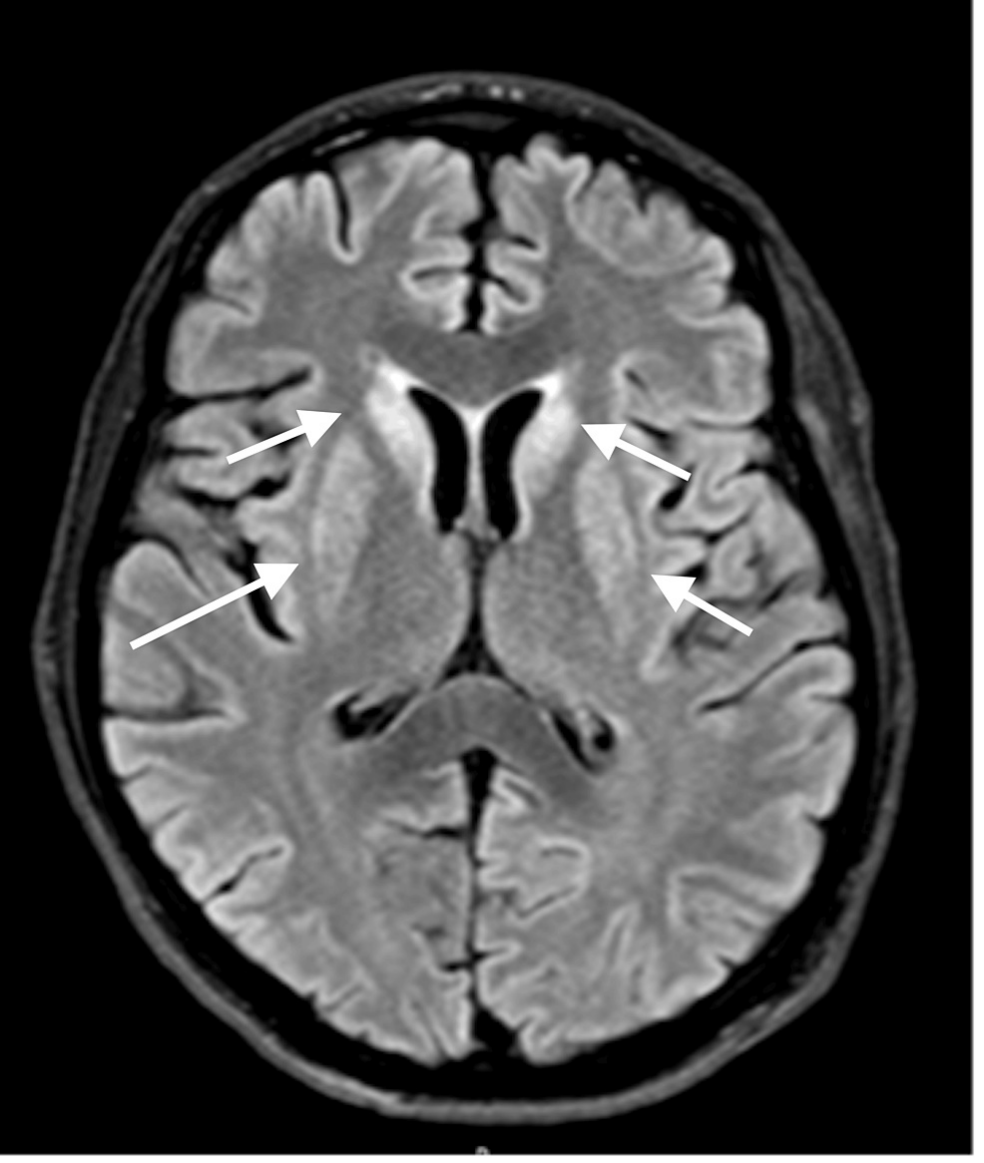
Repeat MRI of the brain with and without contrast, three weeks after admission, revealed T2-FLAIR hyperintensities in the bilateral basal ganglia, consistent with hypoxic ischemic brain injury.
